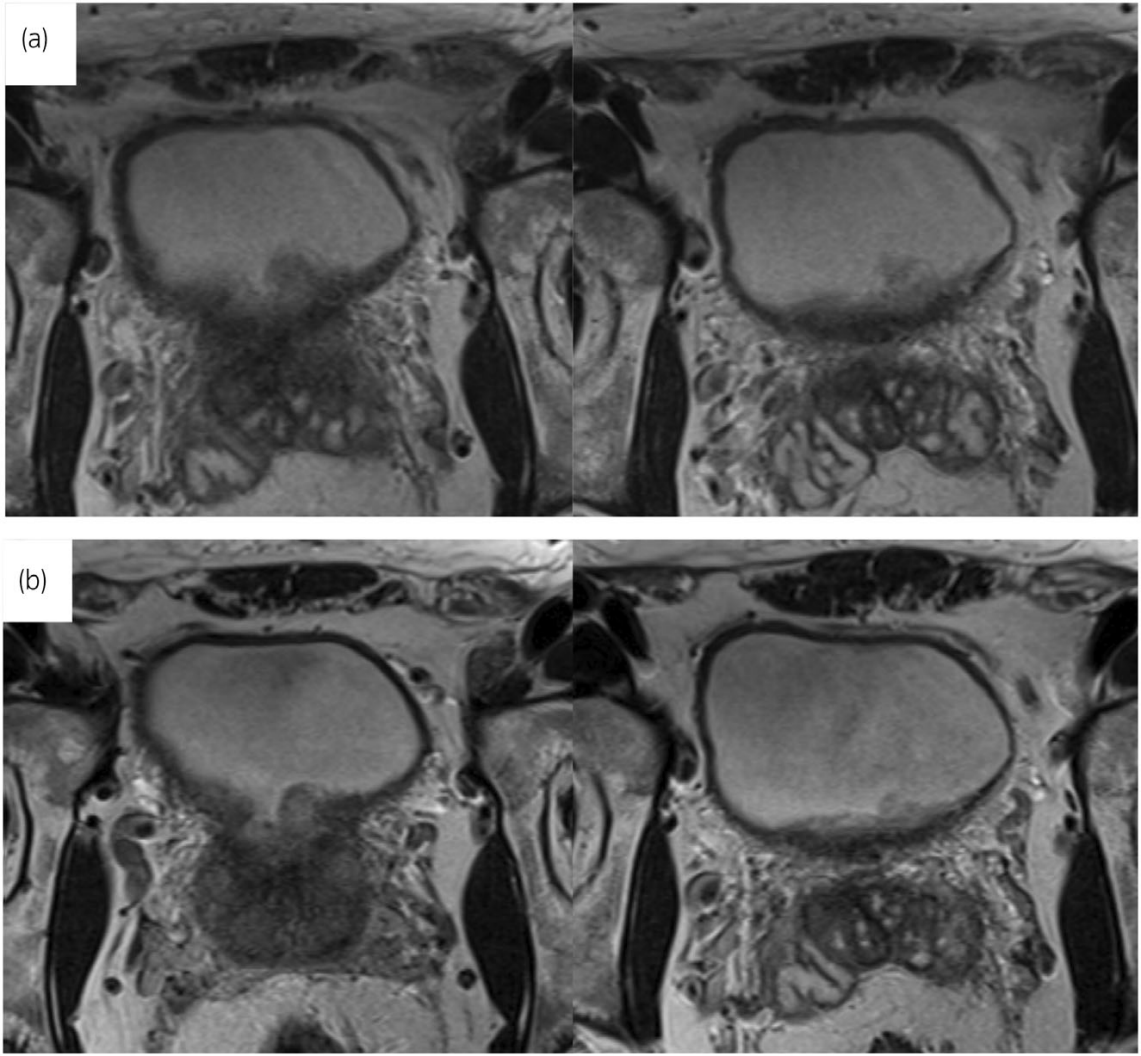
Findings of MRI. (a) Findings of MR before oral administration of COX‐2 inhibitor. T2‐weighted imaging showed a high‐signal thickened bladder mucosa, suggesting proliferative cystitis. (b) Findings of MRI 6 weeks after oral administration of COX‐2 inhibitor. MRI revealed slight tumor shrinkage.
